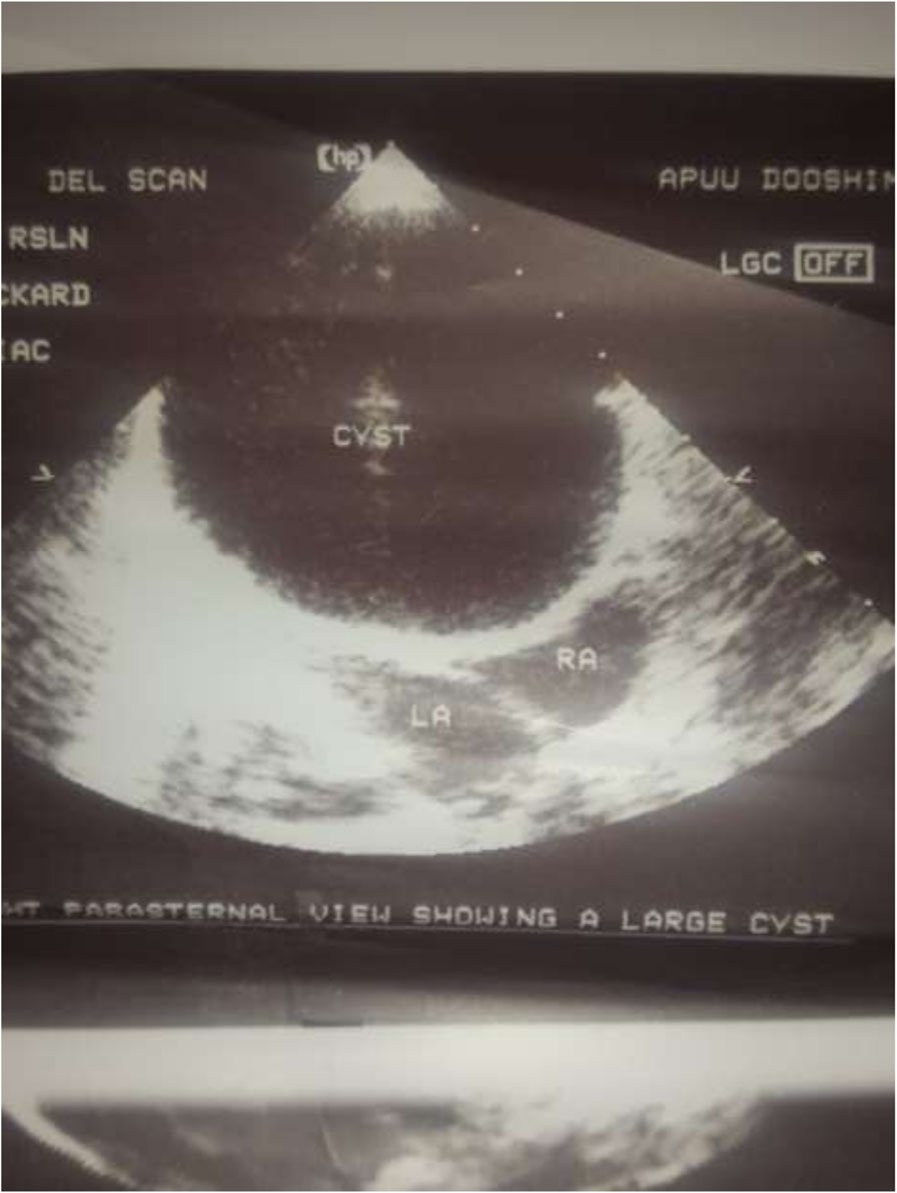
Echocardiography showing the cyst
